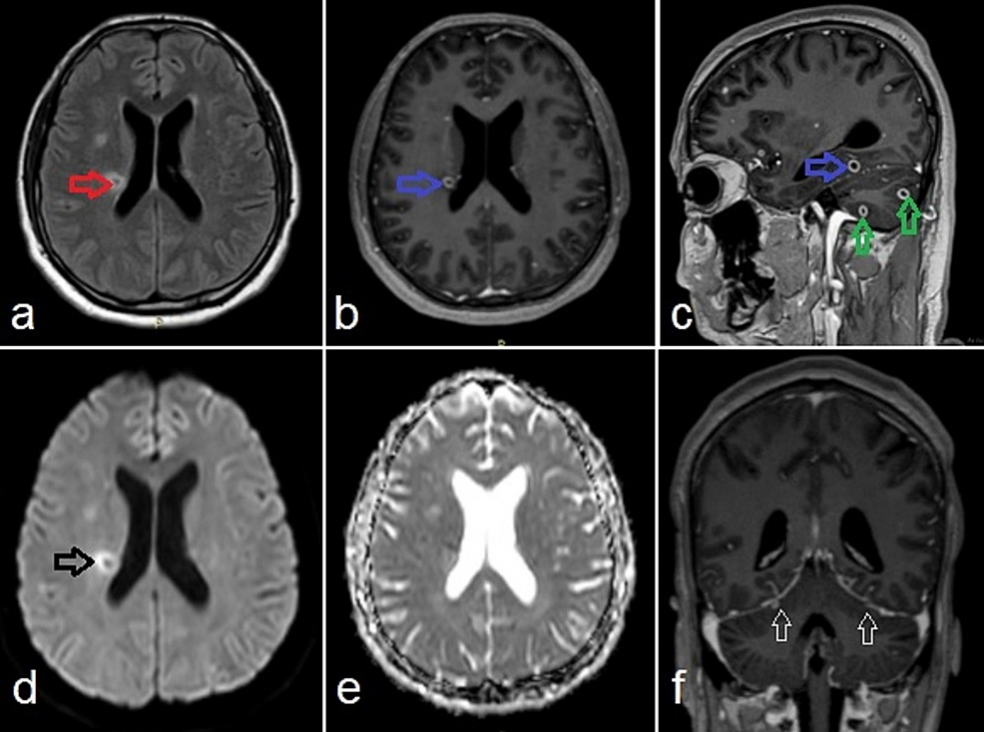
Brain MRI T1WI post-contrast axial (b) and sagittal (c) sections showing multiple ring-enhancing supra and infra-tentorial lesions (blue and green arrows, respectively) (b and c). Their wall appears hyperintense on FLAIR (red arrows in a). These show peripheral ADC (e) high signal (black arrows) with no central diffusion restriction (d) favoring tuberculomas. Furthermore, there is mild nodularity along the tentorium suggestive of meningeal involvement (white arrows in f). T1WI, T1-weighted image; FLAIR, fluid-attenuated inversion recovery; ADC, apparent diffusion coefficient
